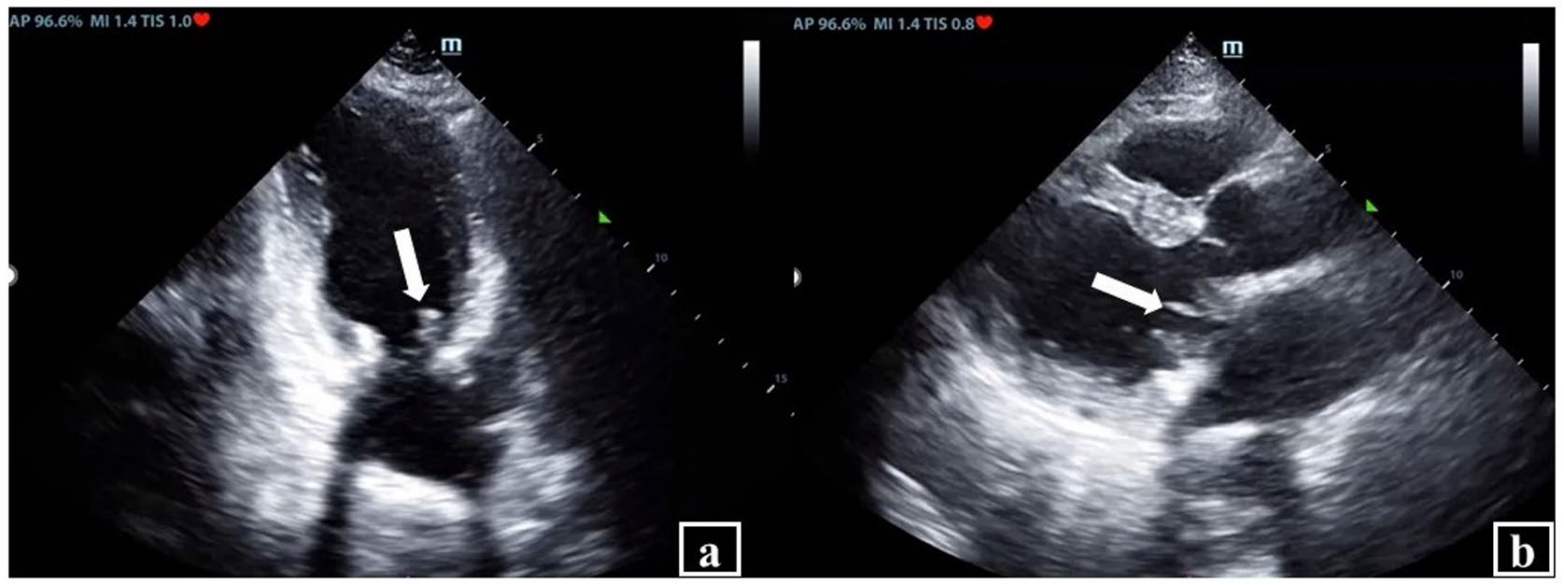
Transthoracic echocardiography (TTE) in *Candida albicans* complex prosthetic valve endocarditis. (a) TTE demonstrated thickened mitral bioprosthetic valve leaflets, a 9 × 5 mm flocculent (arrow). (b) Accompanied by restricted valve opening and incomplete closure (arrow).
